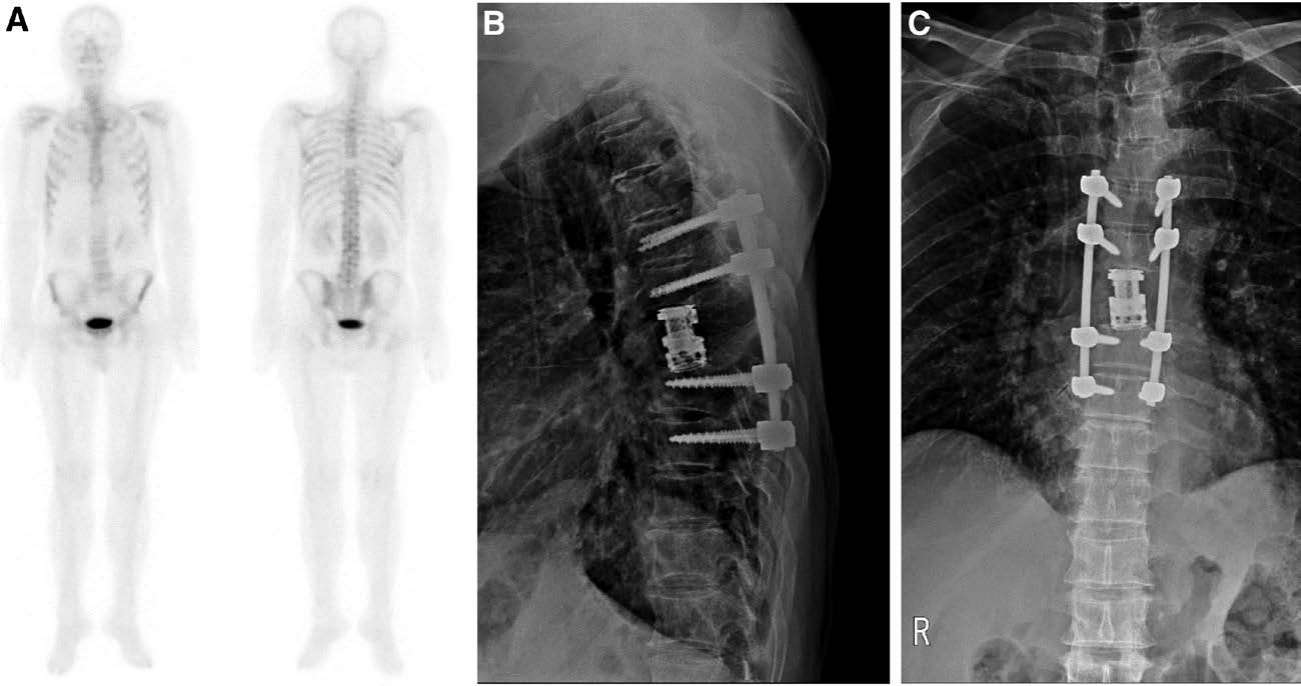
Latest follow-up bone scan (POD 20 mo): A showing no metastasis. Latest follow-up radiographs (POD 18 mo): B and C show well-maintained stabilization.
